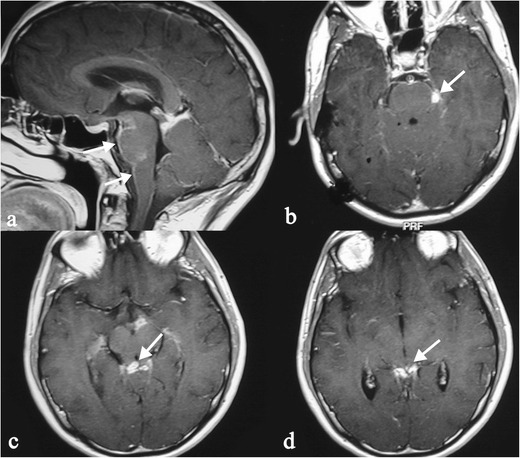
Leptomeningeal localizations of sarcoidosis. Lesions are more visible on enhanced T1-weighted acquisitions, with increased enhancement along the surface of the brainstem (*white arrows* in Fig. 14a–b). Sarcoid nodules are also visible in the superior cerebellar cistern (*white arrows* in Fig. 14c–d)
